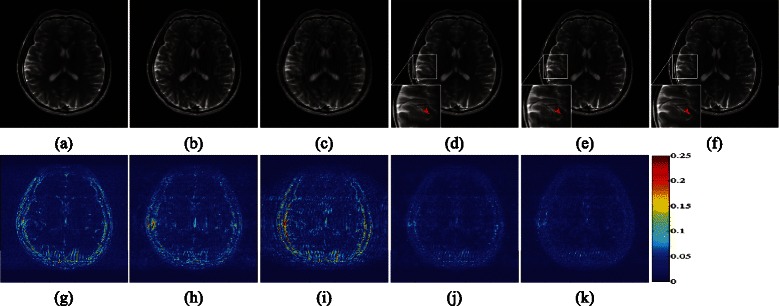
Performance of algorithms under Cartesian undersampling scheme at 35 *%* sampling rate. (a)–(e) Reconstructed images using DLMRI, MRSFLCT based CS MRI, LORAKS, PANO and LSECSMRI, respectively, (f) reconstructed image from fully sampled *k*-space data, (g)–(k) difference images between fully sampled MR image and images in (a)–(e) with gray scale of [0,0.25], respectively. PSNRs of them are 31.61, 32.28, 28.53, 36.33 and 3
